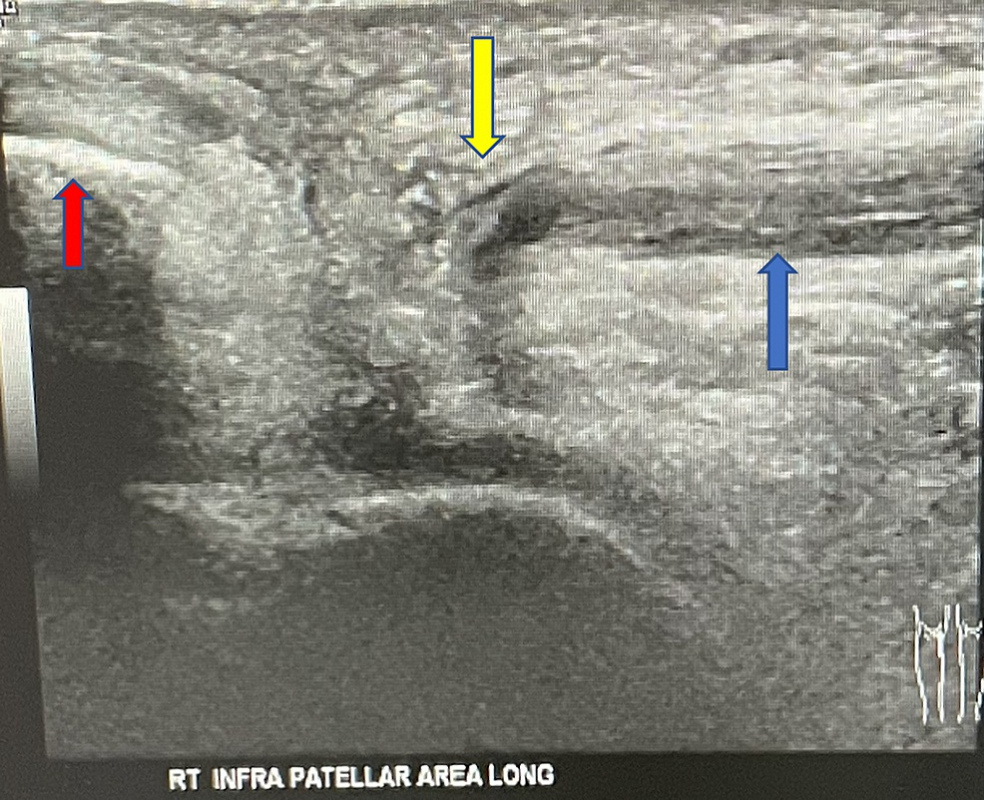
Ultrasound of the right knee showing patellar tendon rupture. Non-visualization of the right patellar tendon (blue arrow) to its attachment at the inferior pole of the patella (red arrow). The area of tendon disruption is indicated by the yellow arrow.
